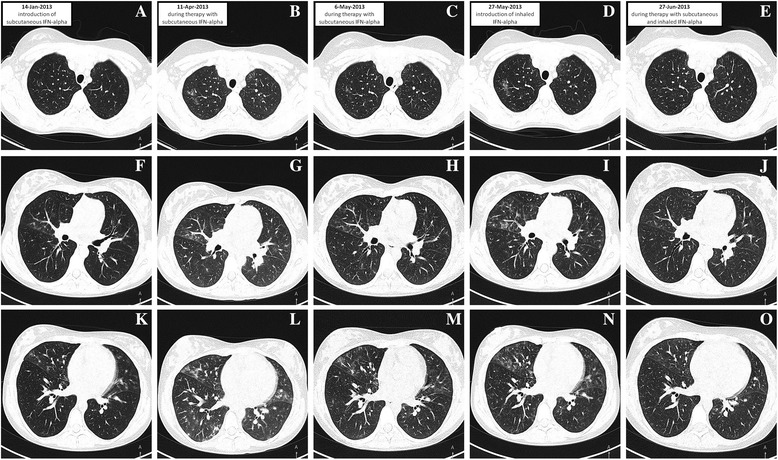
Consecutive CT scans obtained from the patient: AFK – before introduction of IFN-alpha (14-Jan-2013), BGL – during treatment with subcutaneous IFN-alpha (11-Apr-2013), CHM – during treatment with subcutaneous IFN-alpha (6-May-2013), DIN – before introduction of inhaled IFN-alpha (27-May-2013), EJO – during treatment with subcutaneous IFN-alpha and inhaled IFN-alpha (27-Jun-2013)
